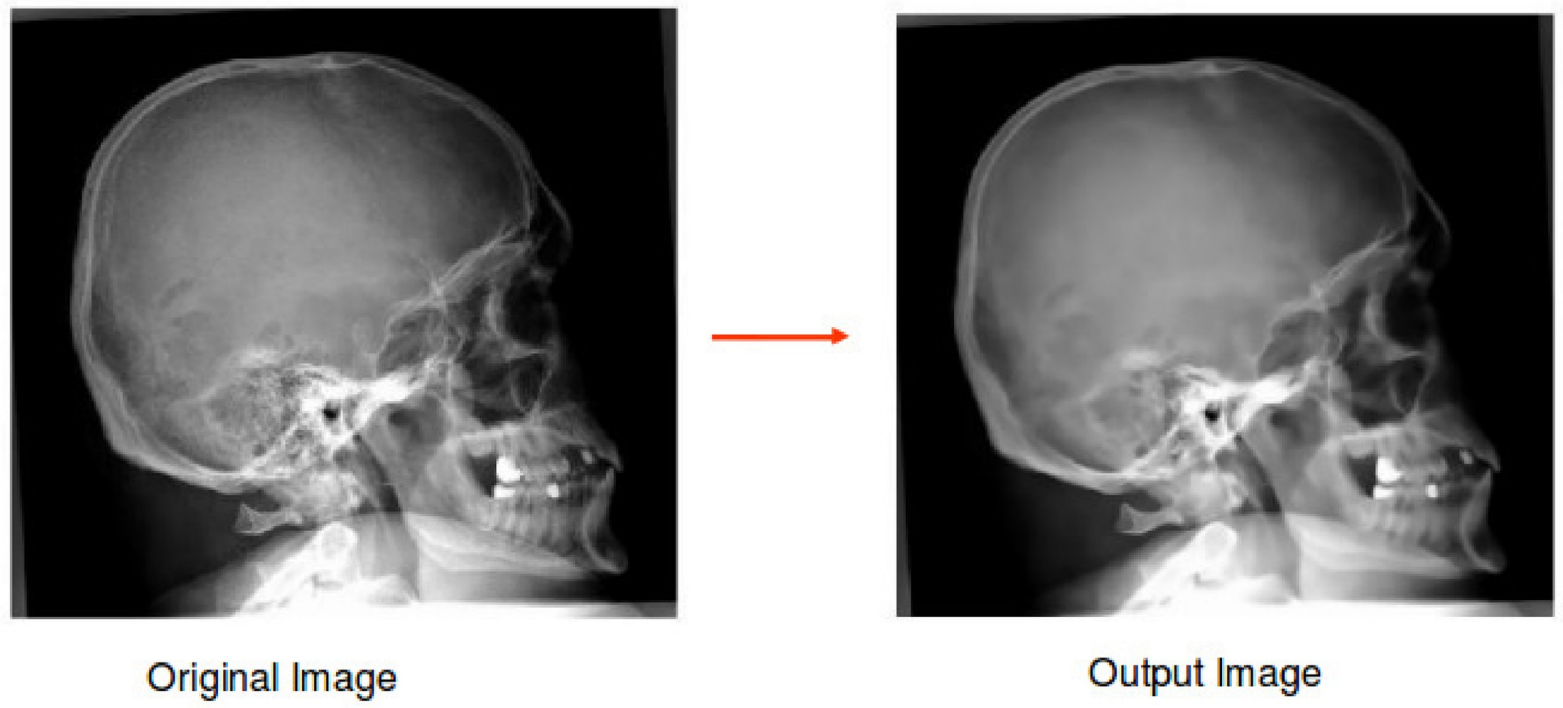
Medical image before and after applying the Non Local Means (NL-MEANS) preprocessing method. The radiograph was randomly chosen from the ImageCLEF 2009 Medical Annotation Task Training Set. Republished from [21] under a CC BY license, with permission from [RWTH Aachen], original copyright [2009].
